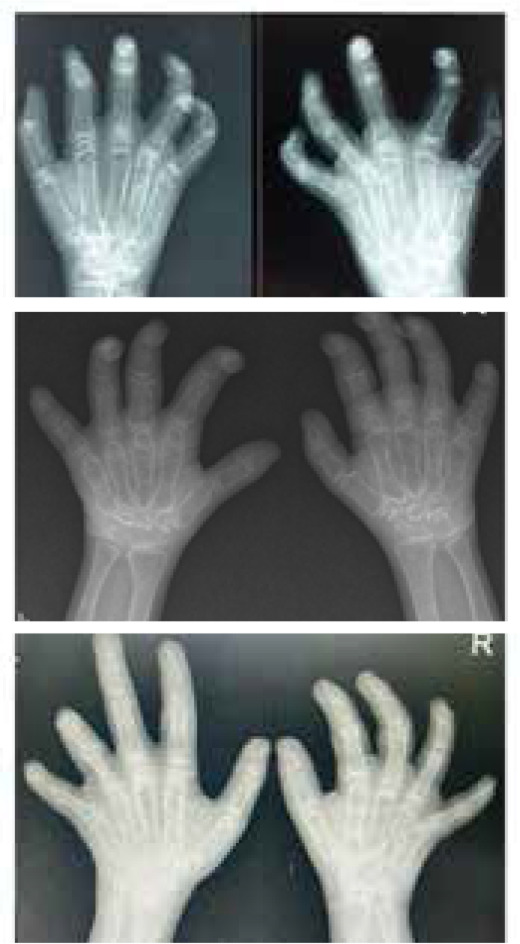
Hand radiographs of children with MONA. (A) P1 at the age of 12 years. (B) P2 at the age of 5 years. (C) P3 at 10 years. well-formed carpals can be seen in the early stages (A) and (C). In the later stages diffuse osteopenia, osteolysis of carpal bones, ankylosis of carpal bones, and erosions at the base of PIP can be seen (B).
